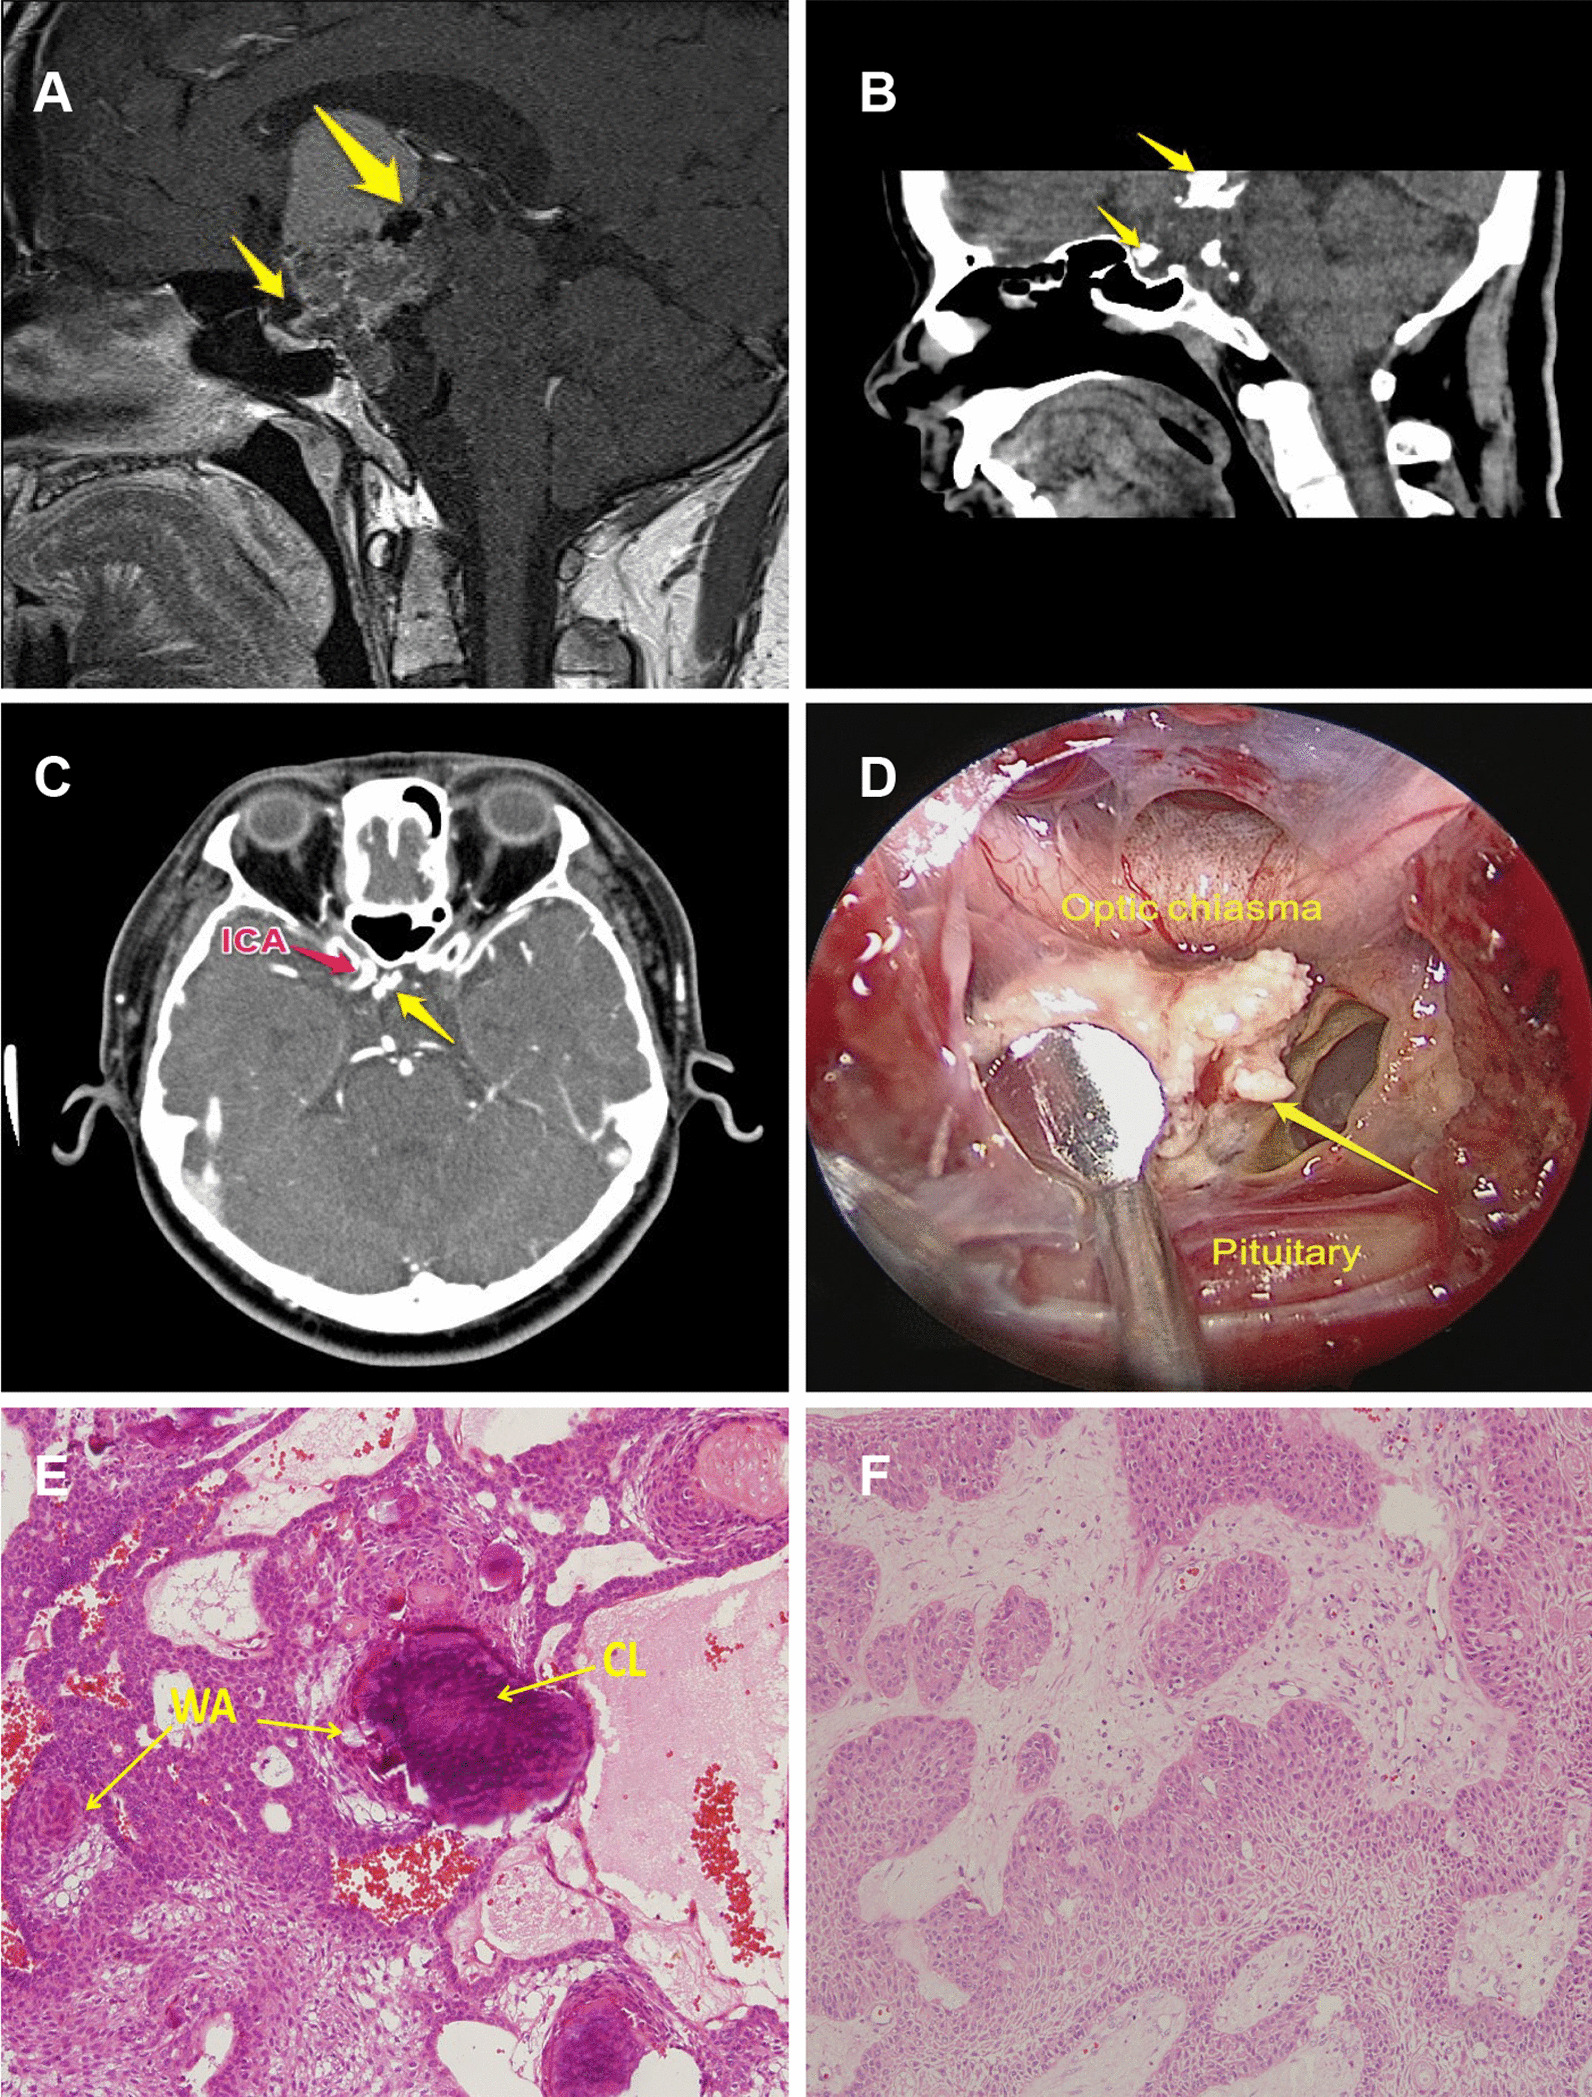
Relationship between calcification of ACP and its surrounding anatomic structures. A, B Sagittal MR and CT scans showing tumor calcifications (yellow arrows: calcification lesions are shown by the low signal in the MR image presented in A and high density in the CT scan presented in B) located above the pituitary gland and below the hypothalamus. C The calcification lesion (yellow arrow) was closely related to the right ICA. D Under the surgical view from the extended endonasal endoscopic perspective, the calcification lesion (yellow arrow) was found to adhere to the optic chiasm and pituitary gland. Surgeons must be very careful when separating the lesion from the surrounding anatomical structures (hypothalamus, ICA and optic chiasm) to avoid serious postoperative complications. (ICA: internal carotid artery). E Calcification lesion (CL) and its precursor structure the whorl-like array cells (WA) were found in adamantinomatous craniopharyngioma sample. We can see a calcification lesion (CL) is arising from its whorl-like array cells (WA) at the central part of this image. F Squamous papillary craniopharyngioma comprising mature squamous epithelia without calcification
